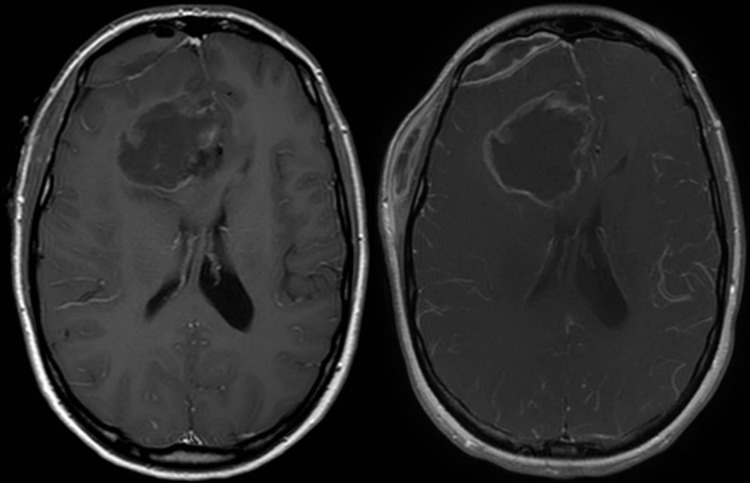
Postoperative T1-weighted MRI with contrast On the left, immediate postoperative axial T1 weighted MRI with contrast. On the right, at presentation axial T1 weighted MRI with contrast showing peripheral enhancement and enlargement of the resection cavity, as well as extra-axial and subgaleal fluid collections with peripheral enhancement. MRI - magnetic resonance imaging.
